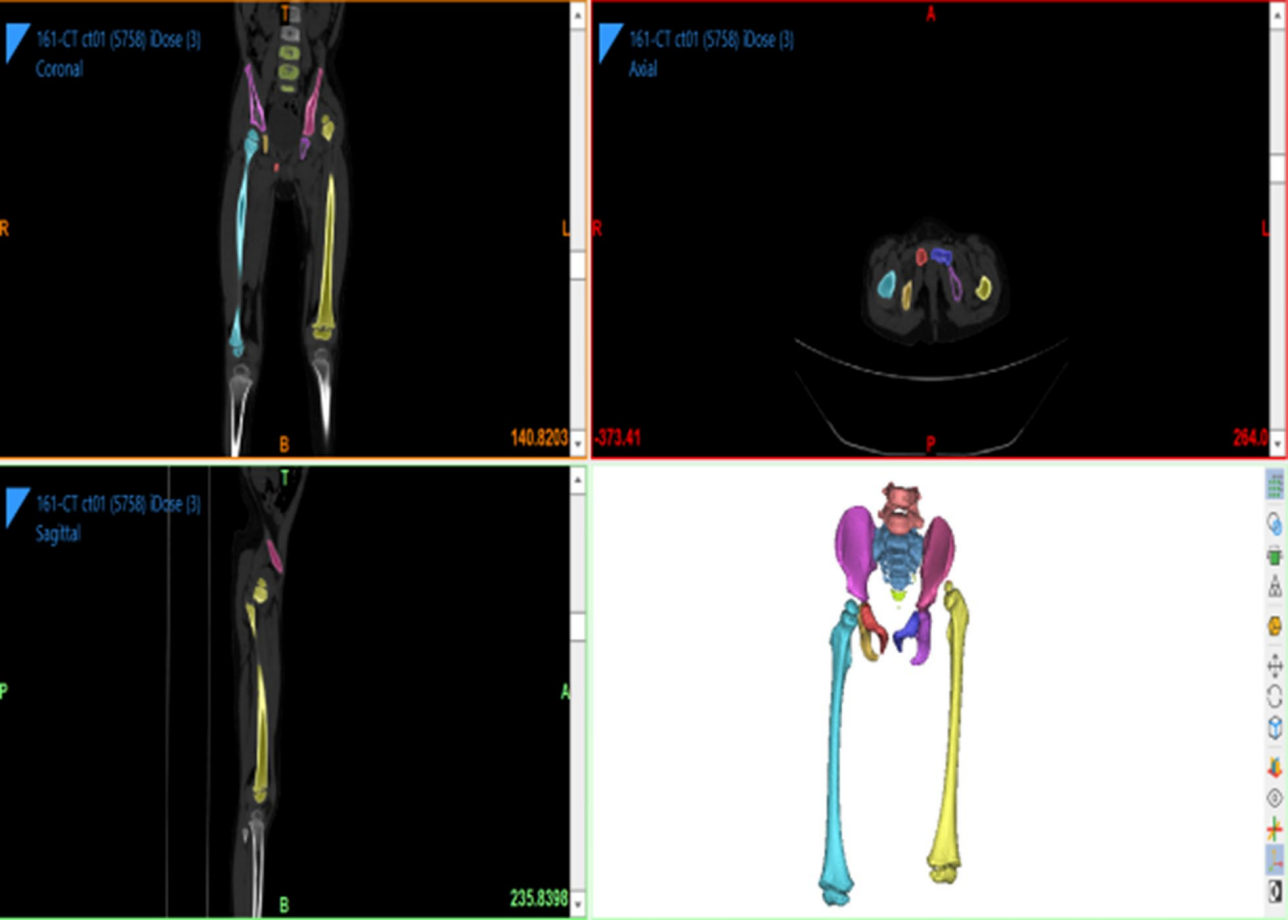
The patient’s CT data are imported into the software, and a mask is created. The original mask is segmented using the region growth command, so that each part of the bone is extracted separately
